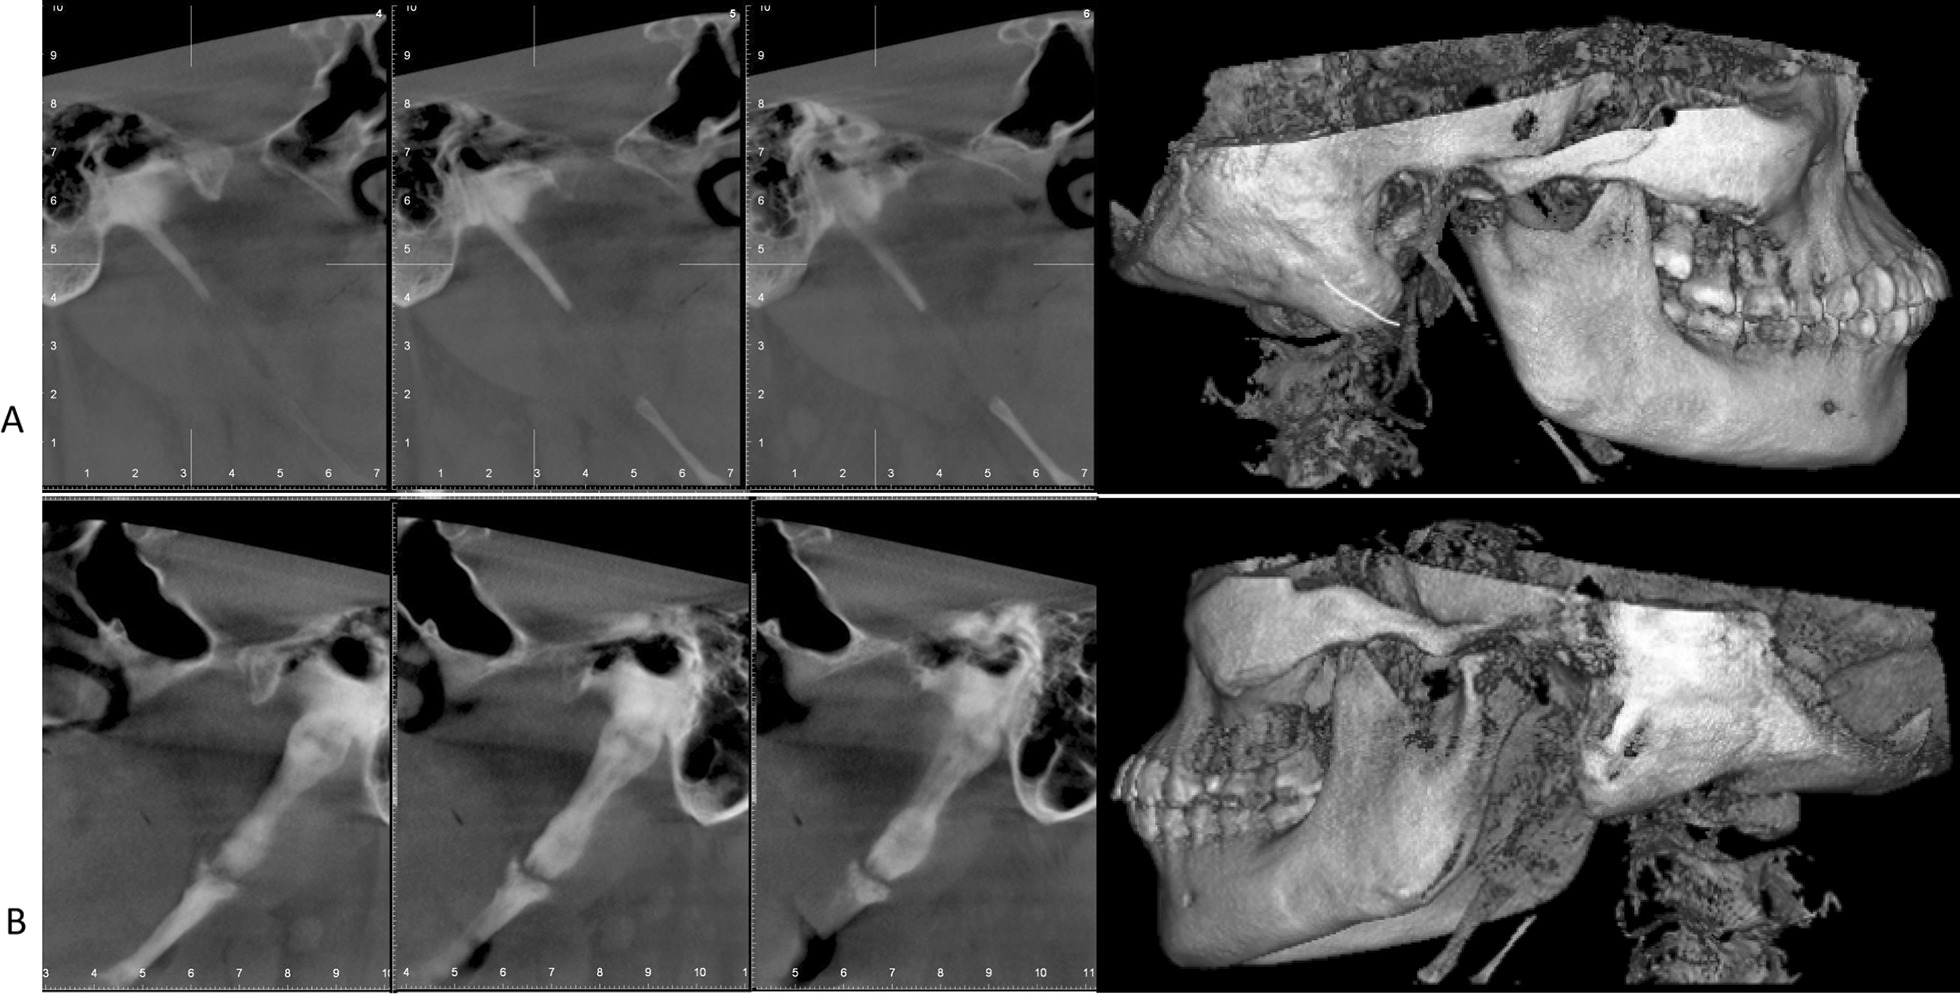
Dental cone beam computed tomography. A Right side: asymptomatic, B Left side, in which the styloid process corresponds to type II of Langlais’s classification; a superior mineralized segment is joined to an inferior mineralized one by a single pseudo-articulation that is located above the inferior border of the mandible
